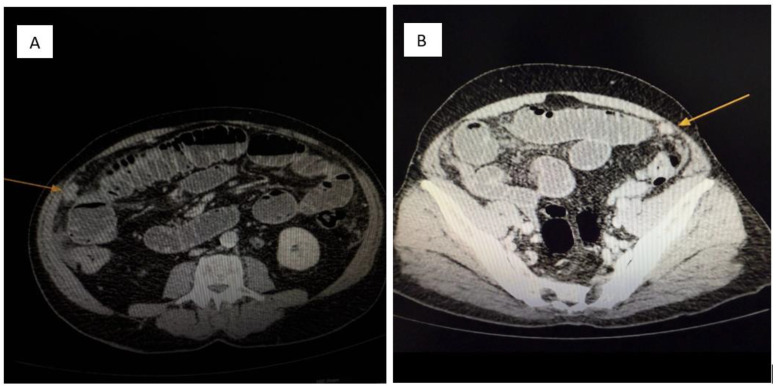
(A,B): Contrast-enhanced abdominal CT. Multiple soft-tissue density nodules (yellow arrow) are diffusely distributed throughout the peritoneal cavity, consistent with metastatic involvement of the parietal peritoneum. The pattern and morphology of these lesions support a diagnosis of peritoneal carcinomatosis (yellow arrow) secondary to a neuroendocrine tumor.
